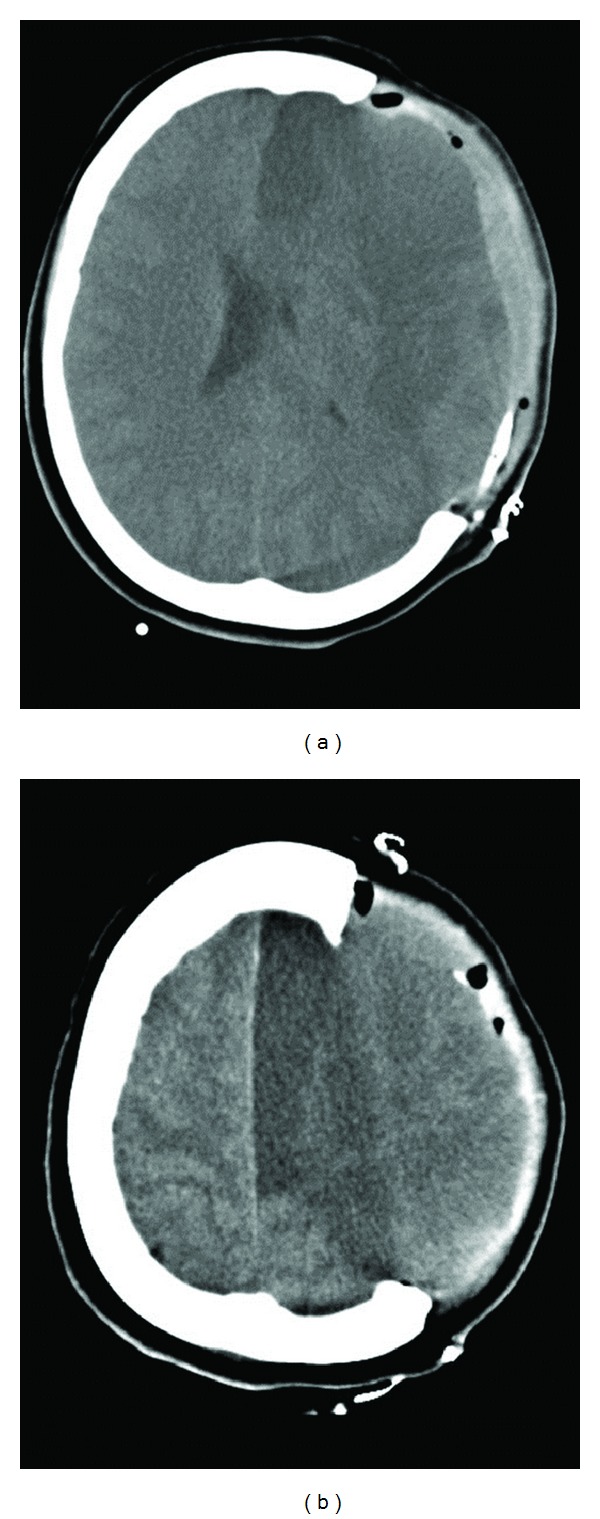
CT scan in a 54-year old female patient who underwent decompressive hemicraniectomy for a large MCA infarction. Note the large bone removal (a) and the left hemisphere occupying this new free space (b).
